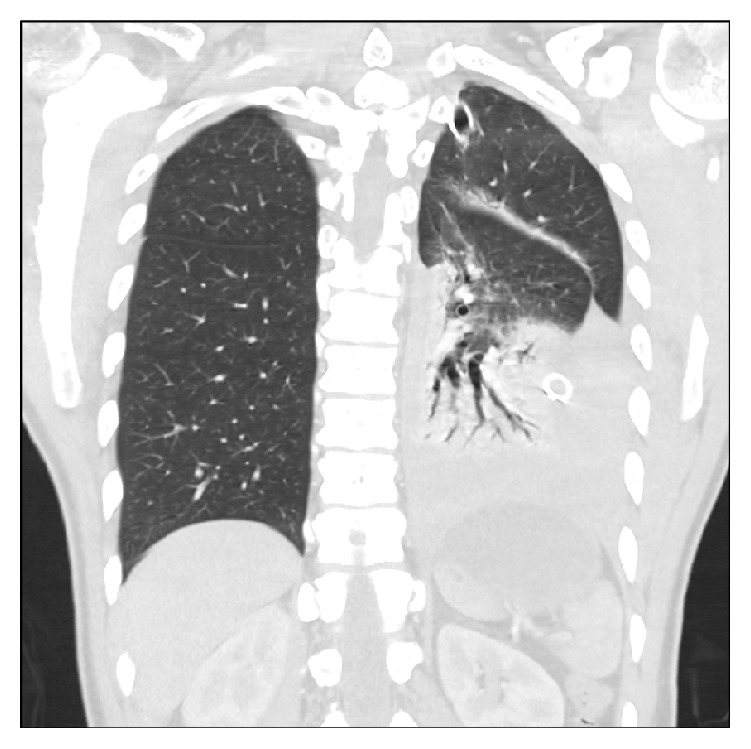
Computed tomography imaging of the chest revealing collapse of the left lower lobe and a large left-sided, high density pleural fluid collection suggestive of hemorrhage continuing into the thoracic cavity despite thoracostomy tube placement.
